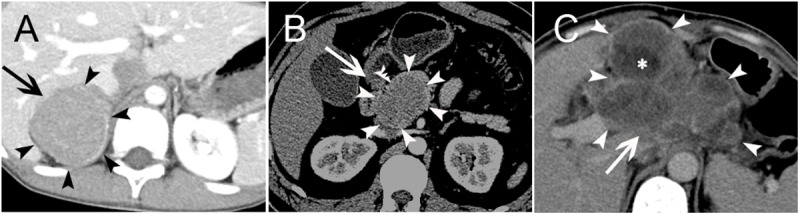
Mild Ring Enhancement. Three PNET cases with masses arising in the right adrenal gland region (A) (Case no. 2), pancreatic head (B) (Case no. 7) and hepatogastric space (C) (Case no. 4) (arrows). Contrast-enhanced CT images showed a mild ring enhancement on portal venous phase (arrowheads), which was more than a half circle surrounding the mass (A-C). The lesions all showed intermediate heterogeneous enhancement. Several patchy cystic areas or septation areas without enhancement (asterisk) could be found in the lesions (C).
